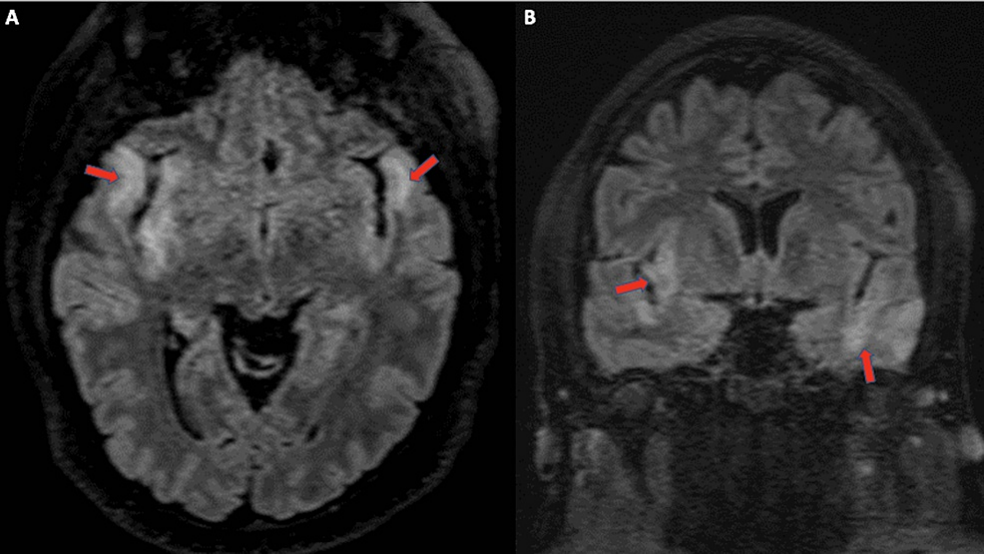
Axial (A) and coronal (B) T2 FLAIR MRI obtained in 2020 Demonstrates hyperintensity of the cortico-subcortical regions of the temporal lobes and right insula (arrows). There was no presence of contrast enhancement or hemorrhage.
